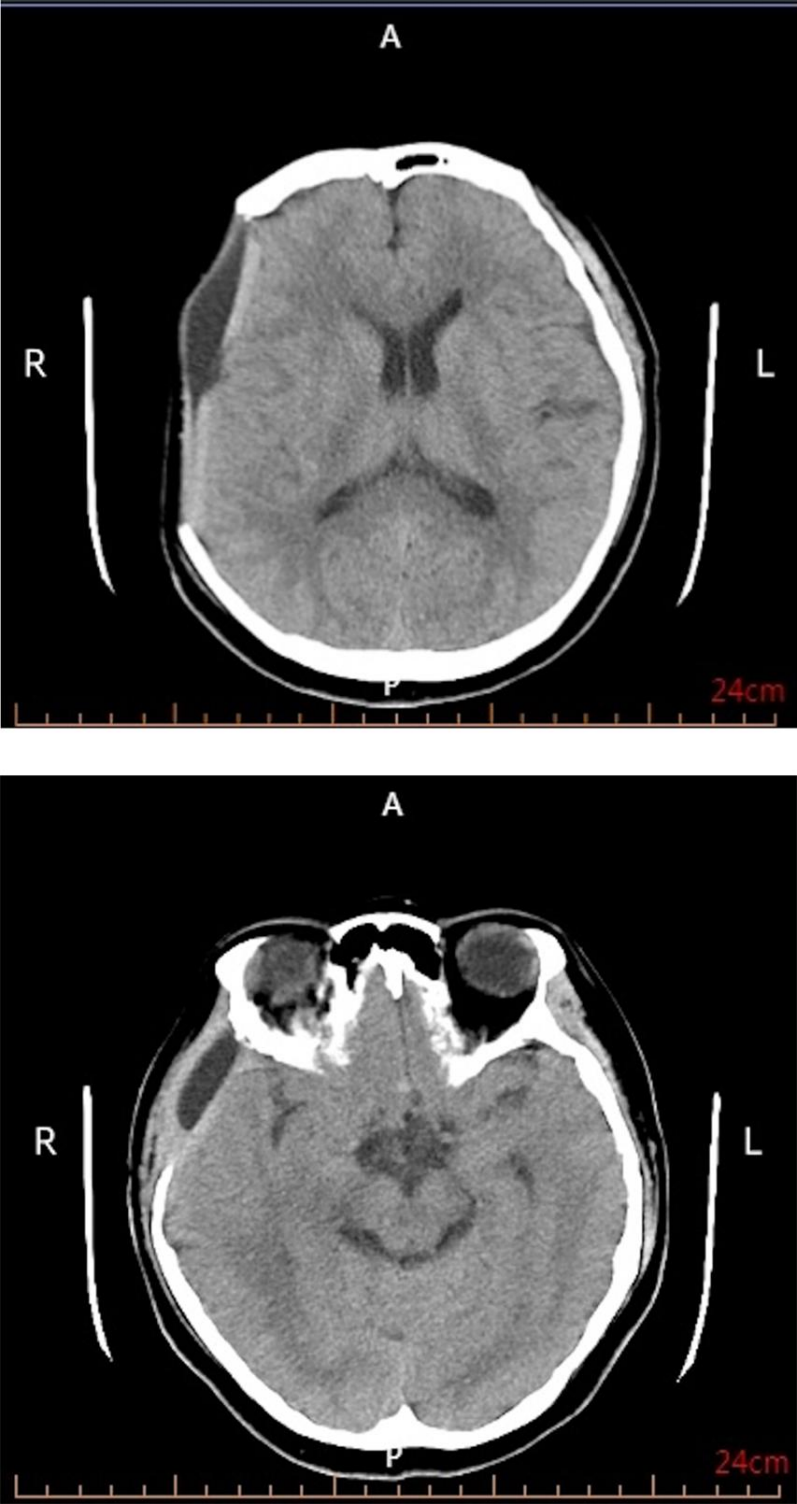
Postoperative follow-up CT. R, right; L, left; A, anterior; P, posterior.
